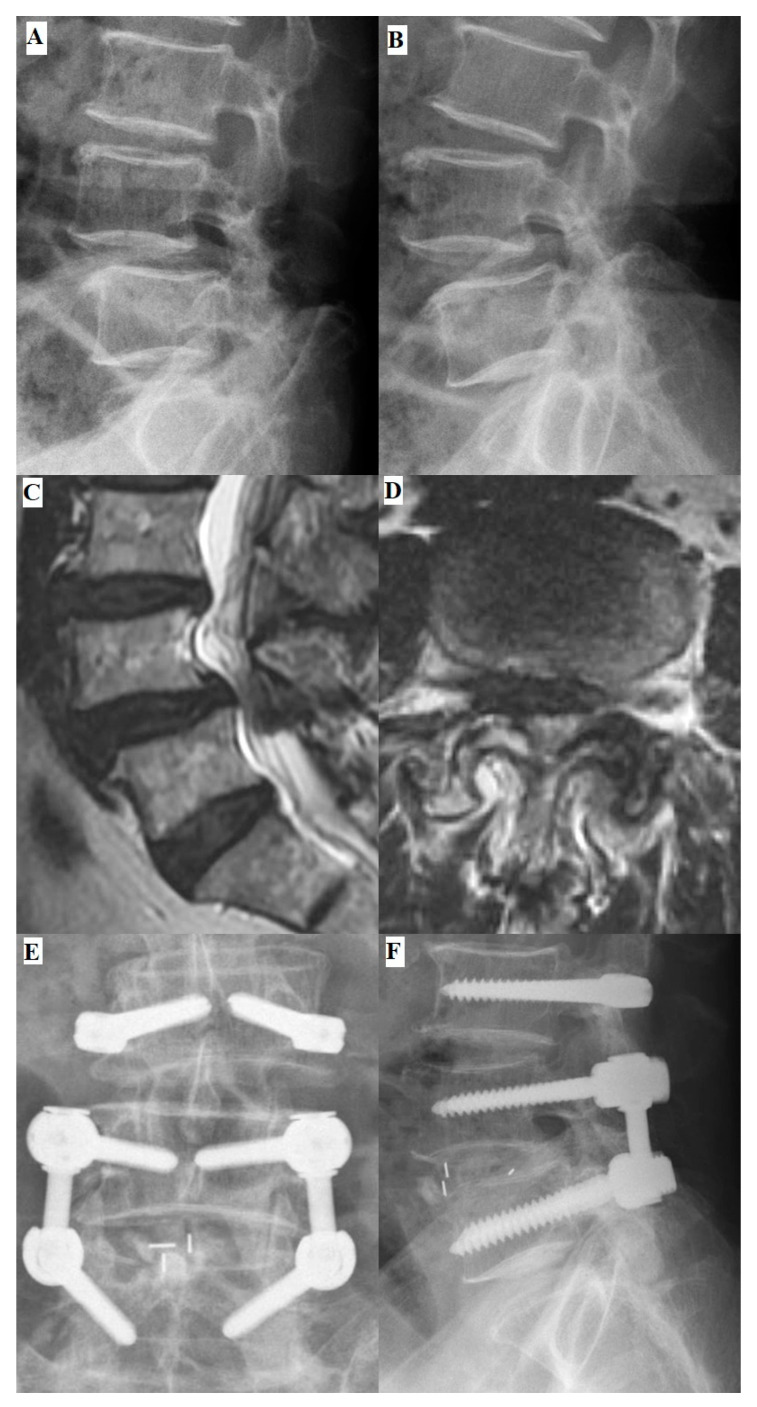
Preoperative and postoperative images of a 71-year-old male patient. The preoperative L-spine flexion (A) and extension (B) radiographs revealed L4–L5 segment spondylolisthesis and the formation of multiple osteophytes. (C) Preoperative magnetic resonance imaging (MRI) T2-weighted image sagittal view showed herniation of the intervertebral disc at the L4–L5 segment. (D) The axial preoperative MRI T2-weighted image revealed severe spinal stenosis with facet hypertrophy at the L4–L5 segment. Postoperative anterior–poster (AP) view (E) and lateral view (F) images revealed well-instrumented implantations and promising correction of the spondylolisthesis.
